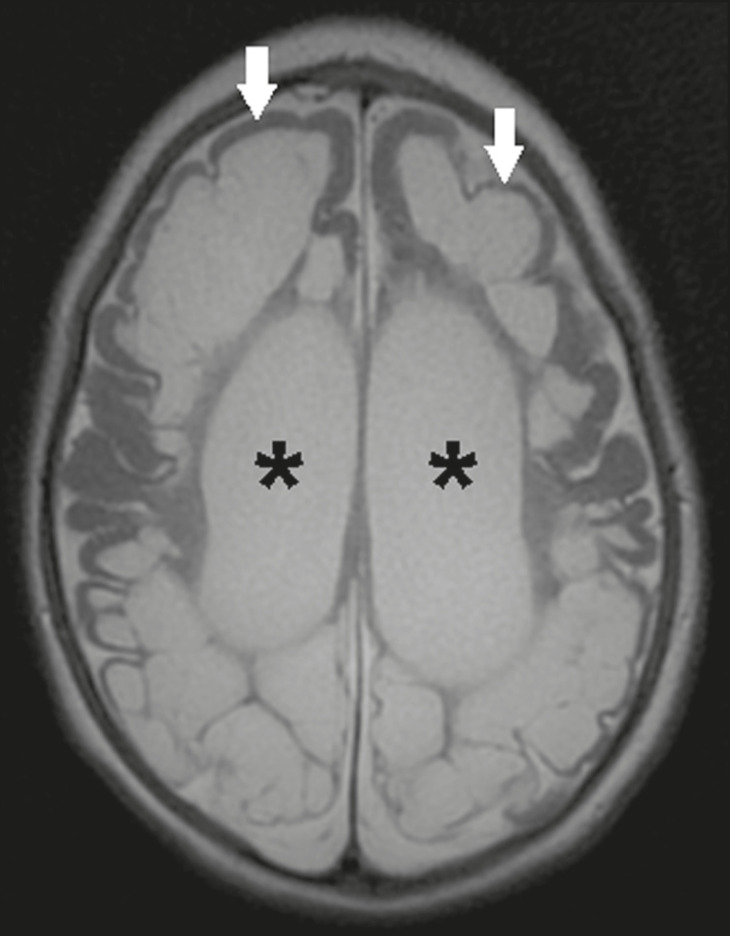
Neonatal hypoxic-ischemic encephalopathy. Axial T2-weighted MRI showing multiple cysts of varying sizes, with intensity similar to that of the CSF, distributed bilaterally in the white matter (arrows). Note the dilatation of the lateral ventricles (asterisks), secondary to involvement of the cerebral parenchyma.
